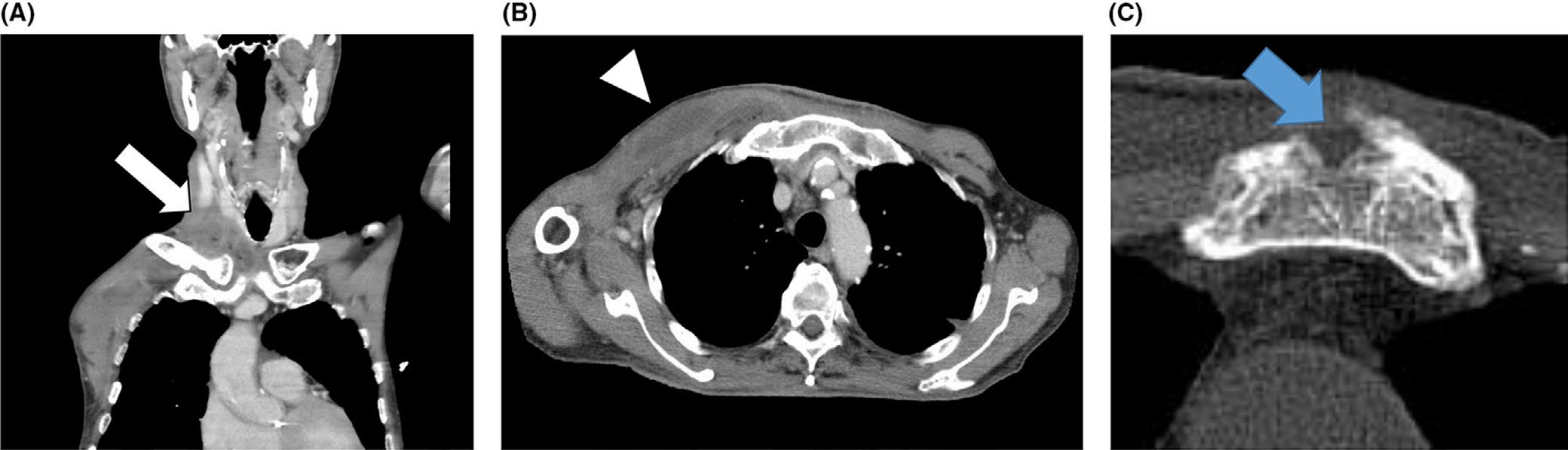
A, Contrast‐enhanced CT scan upon admission showing the coronal section of a neck abscess (white arrow). B, Transverse section of a chest wall abscess (white arrowhead). C, Old sternal fracture with osteolytic change (blue arrow)
